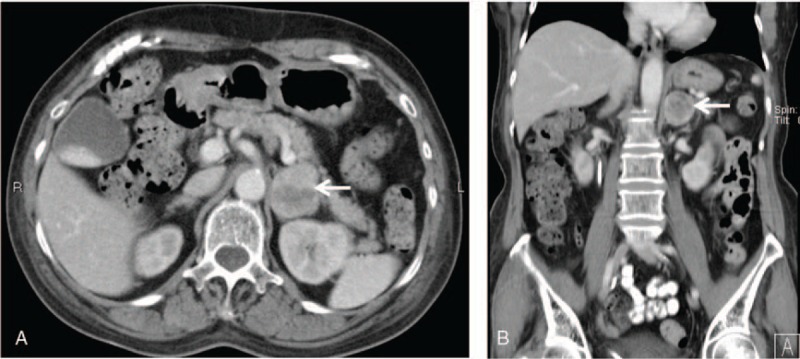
Abdominal computed tomographic scan showing a 3.9 × 3.4 × 3.0-cm well-defined heterogeneous enhanced tumor located in the left adrenal glands.
